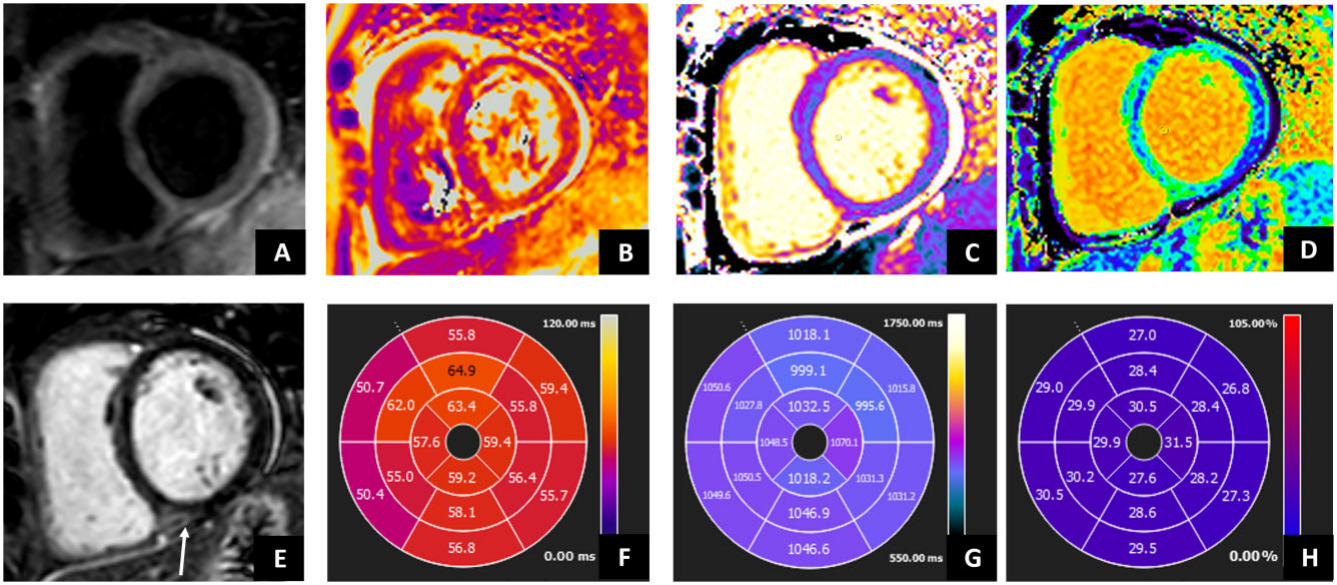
Cardiac MRI in patient with anti-synthetase syndrome. Cardiac MRI shows ventricles with normal volumes and function. STIR images showed (A) absent focal oedema, but subtle diffuse oedema as showed by (B) T2 mapping and (F) AHA bullseye (global value 58 ms; normal value ≤50 ms). (E) Late gadolinium enhancement showed mid-wall hyperintensity in the basal posterior septum, associated with normal native T1 mapping (C and G) (1037 ms; normal value <1045 ms) but increased ECV values (D and H) (29%, normal values <27%), as for mild increase in interstitial fibrosis. Moreover, mild pericardial effusion was evident without significant increase in the pericardium thickness. AHA: American Heart Association; STIR: Short-tau Inversion Recovery; ECV: extracellular volume
